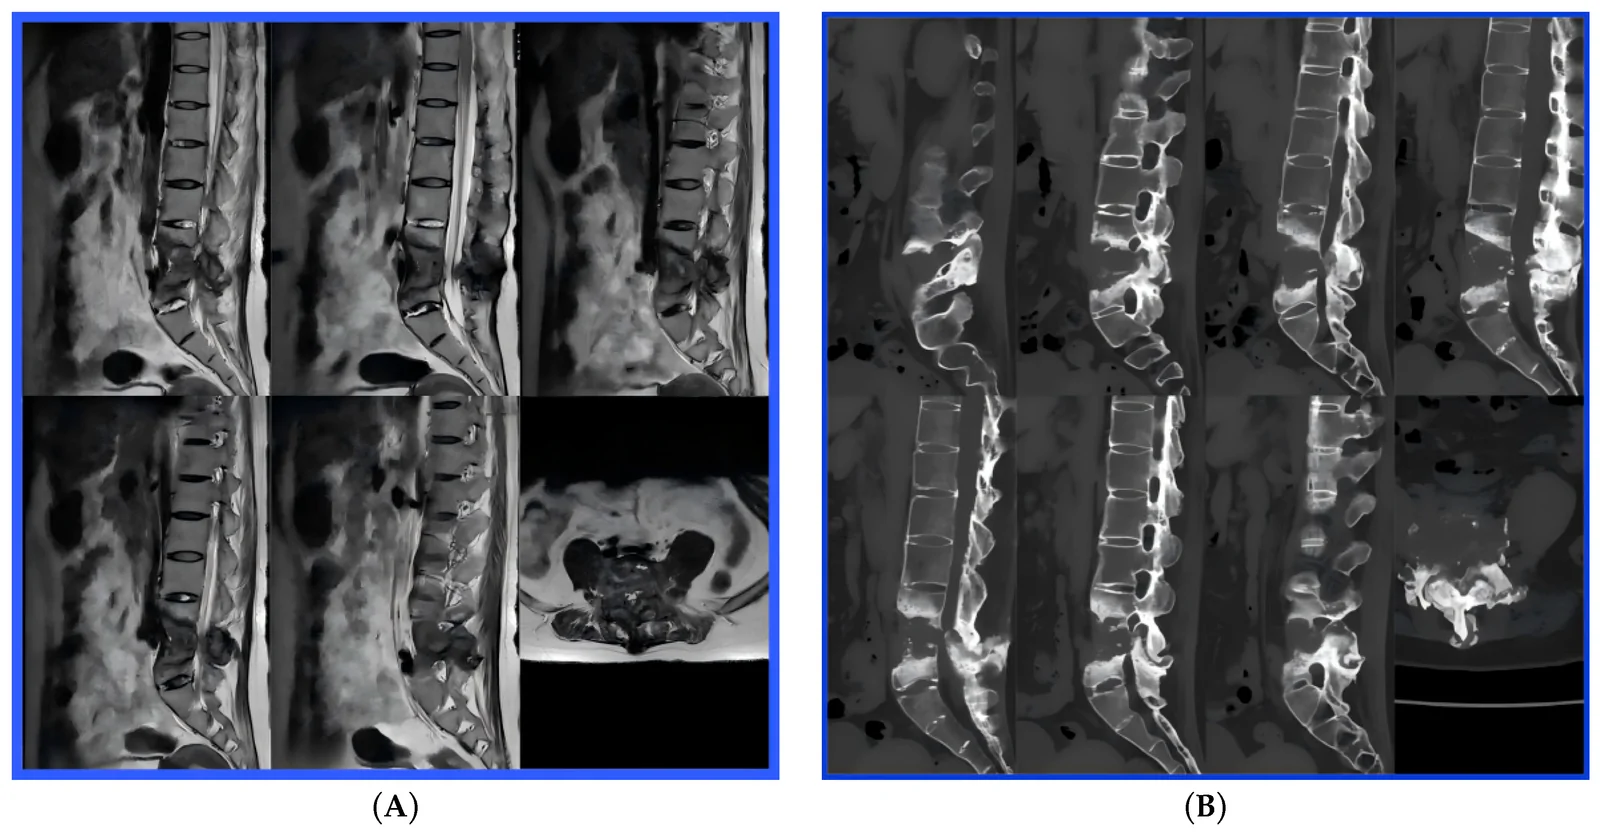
Preoperative advanced imaging. (A) Computed tomography demonstrates bilateral L4/5 pedicle fractures, anterior column bone destruction/resorption, and pseudarthrosis. (B) Magnetic resonance imaging demonstrates adjacent vertebral marrow edema and compression of the left L5 nerve root.
